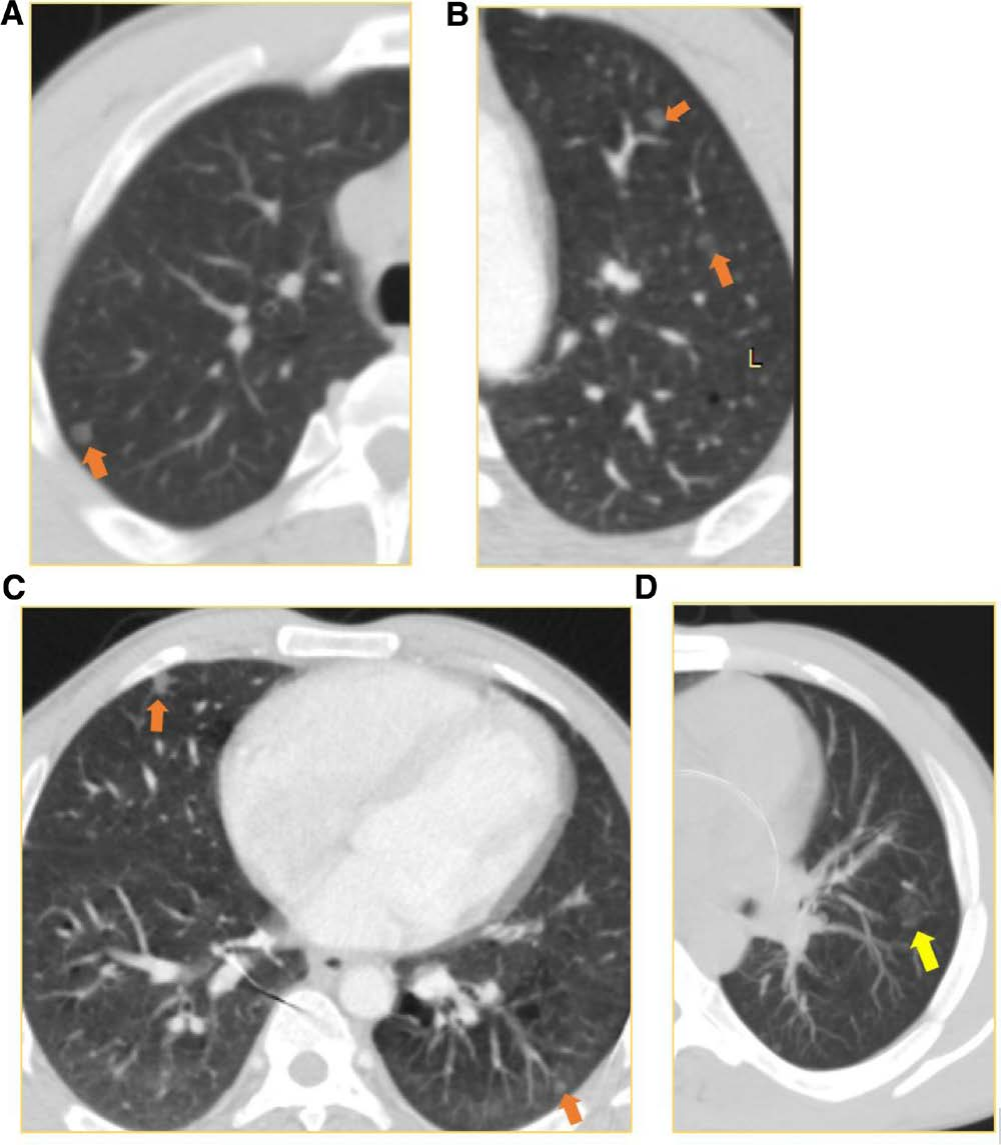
HRCT of the Lung (case-1): Multiple Small (≤ 5mm) well defined randomly distributed ground glass nodules in both lungs (orange arrows in A–C and yellow arrow in D). The characteristics and distribution of the nodules is suggestive of multifocal micronodular pneumocyte hyperplasia (MMPH). There are cystic parenchymal changes in both lungs (B, C).
